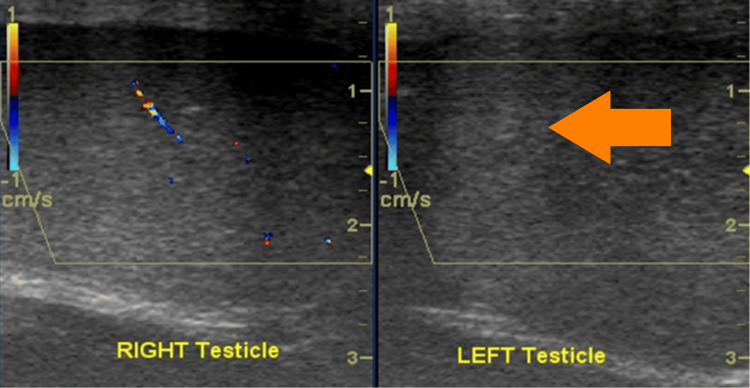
Loss of flow within the left testicle shown on color Doppler US (orange arrow).
